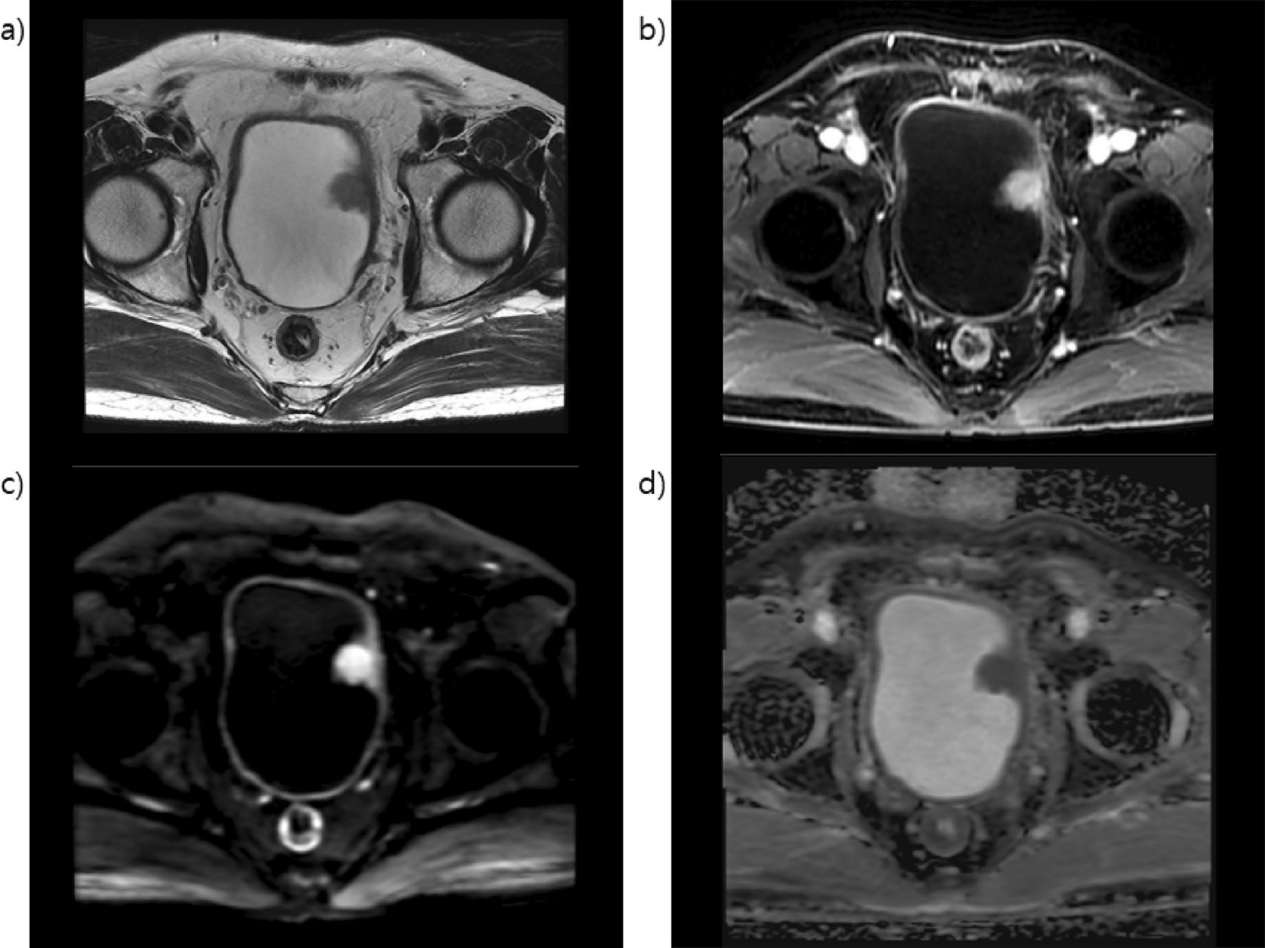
Magnetic resonance images of equivocal muscle invasive bladder cancer (a) T2-weighted image in the left lateral aspect of the bladder wall showing exophytic tumor without high SI thickened inner layer but with no clear disruption of low SI muscularis propria. (b) Early enhancement of muscularis propria but with no clear disruption of low SI muscularis propria. (c) Diffusion-weighted imaging native image and (d) apparent diffusion coefficient map showing muscularis propria with continuous intermediate SI but with no clear disruption of muscularis propria. The muscle layer invasion of tumor is equivocal.
